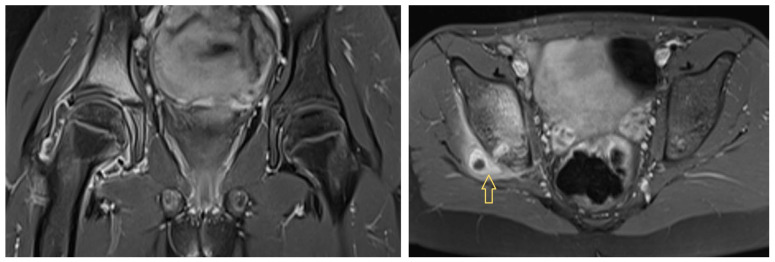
Initial magnetic resonance image of Patient 1 showing coxitis with a fluid path into the gluteal soft tissue. Arrow shows to the fluid path.
